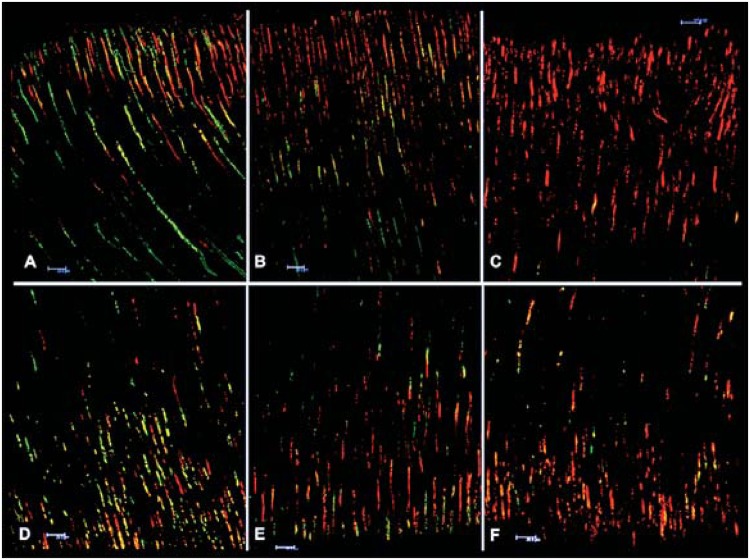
Representative images of bacterial viability in superficial (A, B and C) and deep (D, E and F) dentine obtained by confocal laser scanning: A, D (control group), B, E (without ultrasonic activation of the sealer) and C, F (with ultrasonic activation). Red colour denotes dead bacteria and green denotes live bacteria
